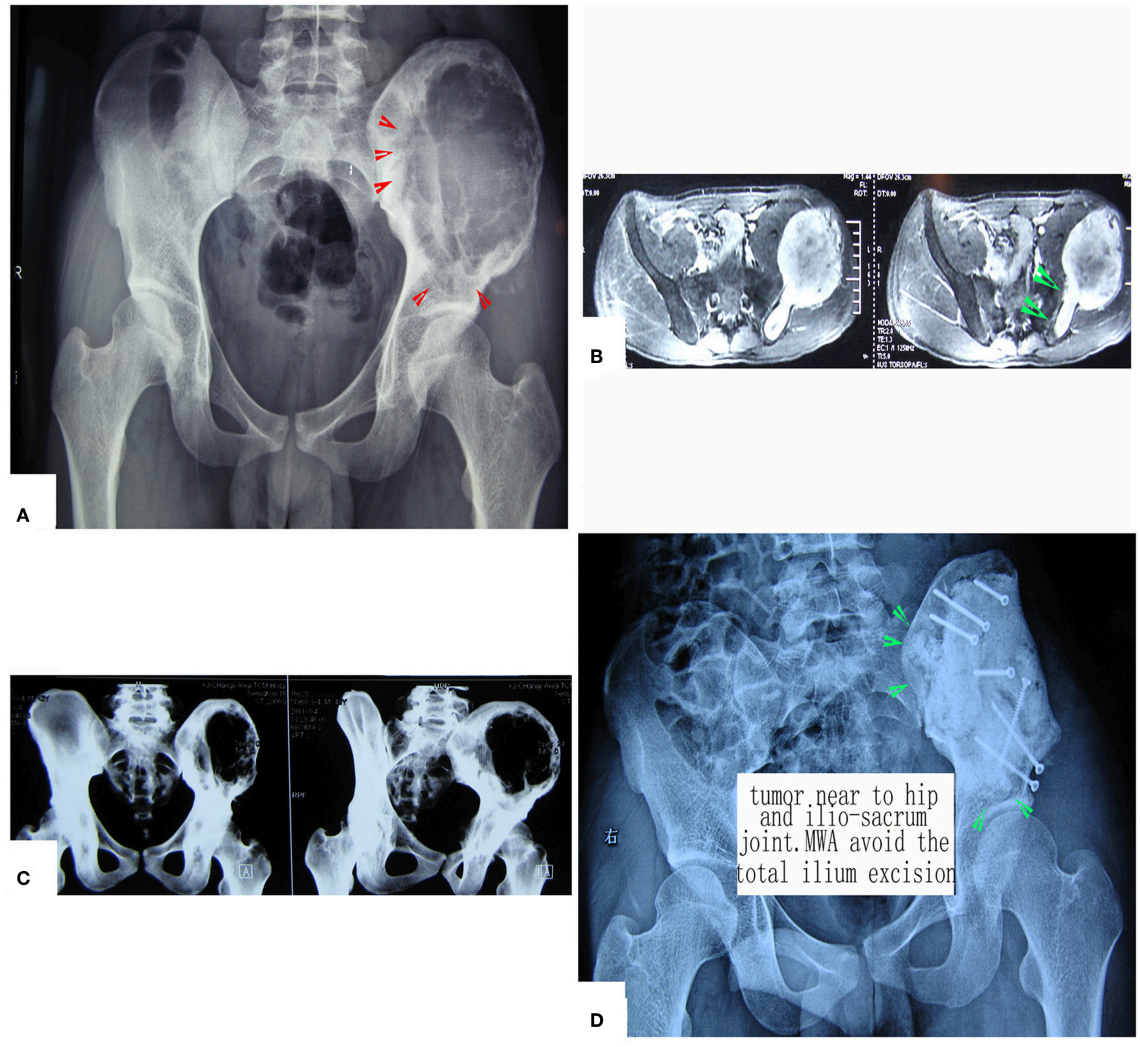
Treatment of a patient with pelvic mesenchymal sarcoma close to the hip joint. (A–C) X-ray and CT scan. Images show a large osteolytic lesion near the hip joint. (D) X-ray after the operation. After MWA, the defect was reconstructed by long screws into the residual ilium bone, and a mixture of cement and morselized allograft bone chips was used to make an artificial pelvic “wall.” The hip joint was reserved completely. The patient was followed up for 7 years, and his hip function is pretty good.
